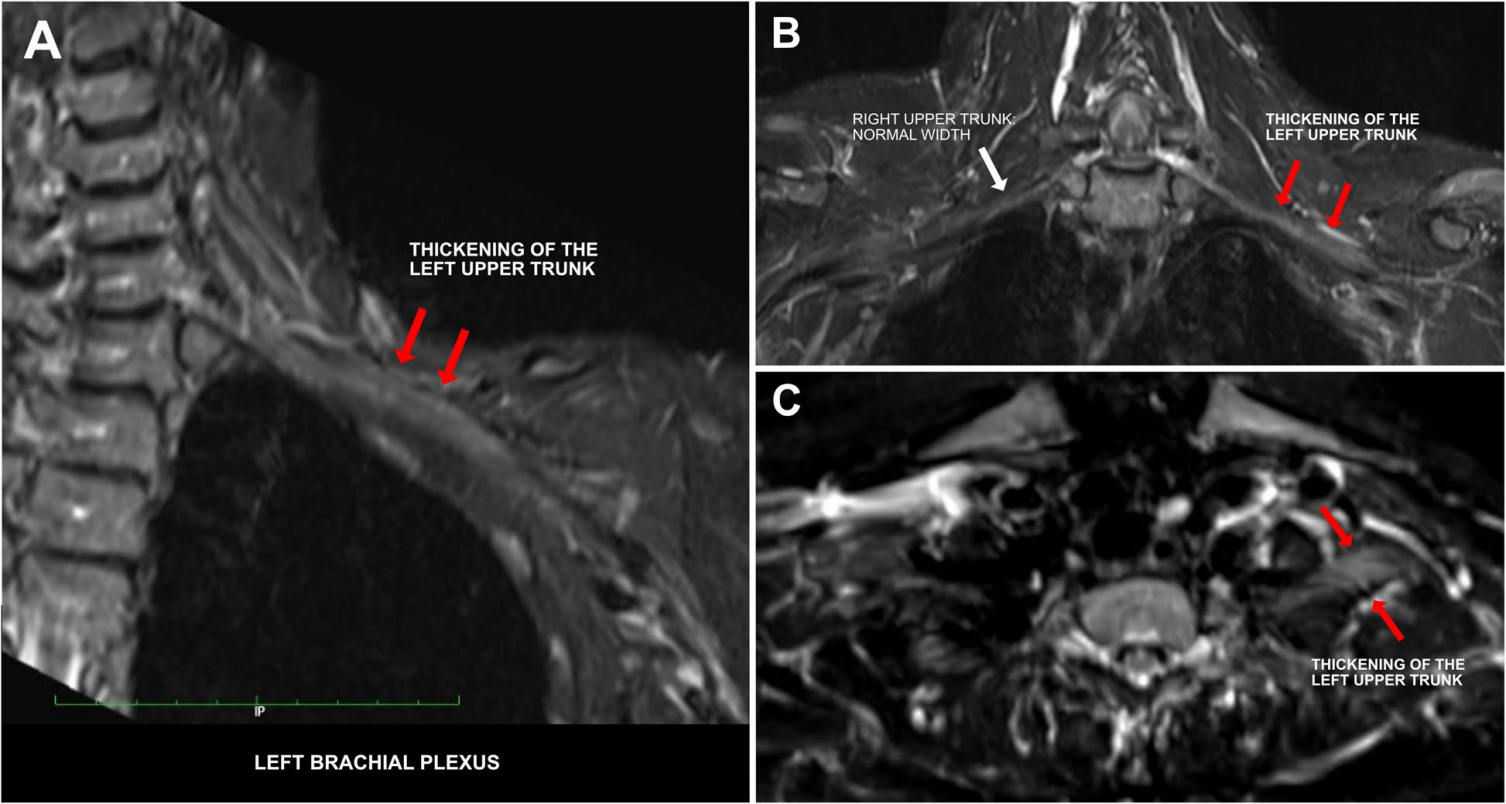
(A-B-C): T2-weighted short-tau inversion recovery (STIR) MRI sequences showing corresponding hyperintensity and thickening of the left upper trunk
